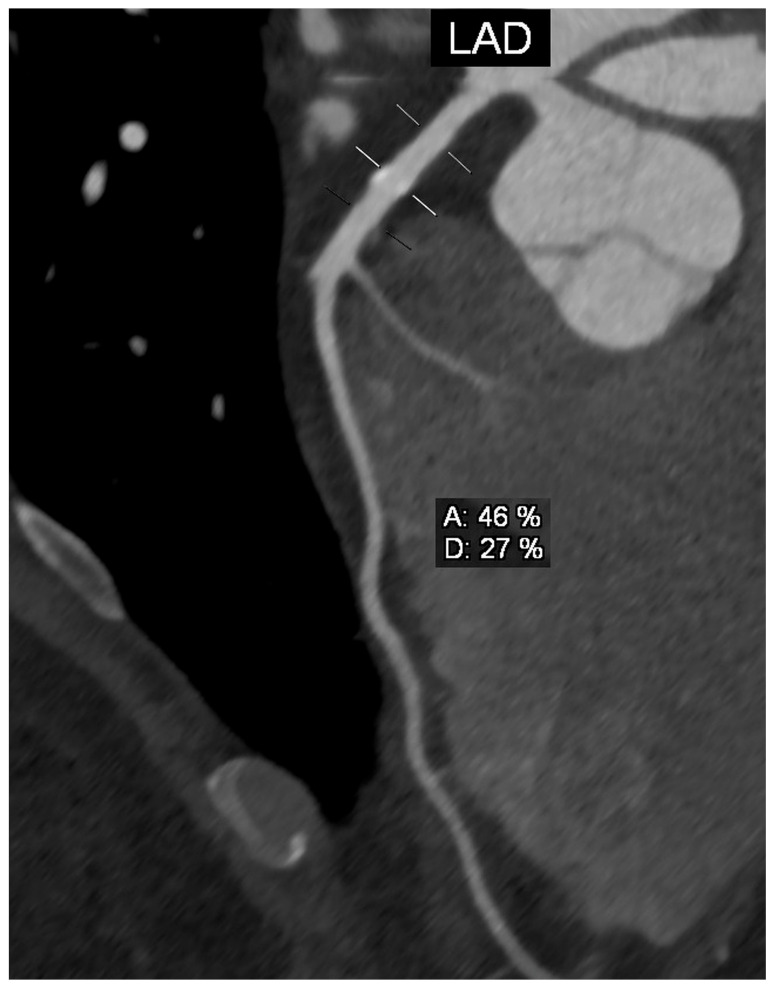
Cardiac computed tomography angiography. Angiographic phase. Curved planar reconstruction (CPR). Left anterior descending (LAD) artery—short-section stenosis of the vessel by 25–50%. Three lines on the course of the vessel: a reference line above the stenosis, a line at the level of the stenosis and a reference line below the stenosis. A—% of stenosis calculated based on the perpendicular cross-sectional area of the vessel, D—% of stenosis calculated based on of the diameter of the vessel.
